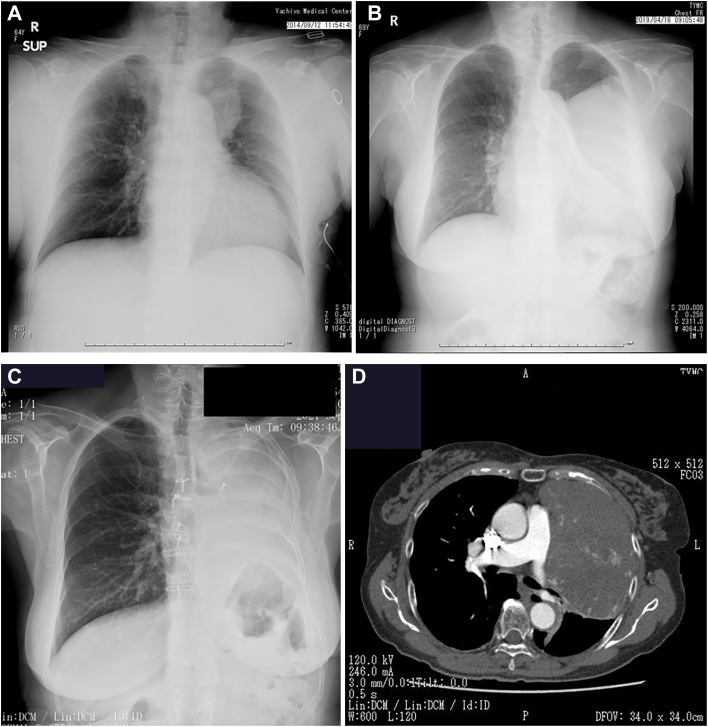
Chest radiographs and computed tomography. (A) Initial chest radiograph showing an anterior mediastinal lesion on earlier evaluation. (B) Chest radiograph on current presentation showing a massive left thoracic opacity with mediastinal shift. (C) Postoperative chest radiograph following left pneumonectomy. (D) Axial contrast-enhanced computed tomography demonstrating a large mass occupying the left hemithorax with mediastinal displacement.
